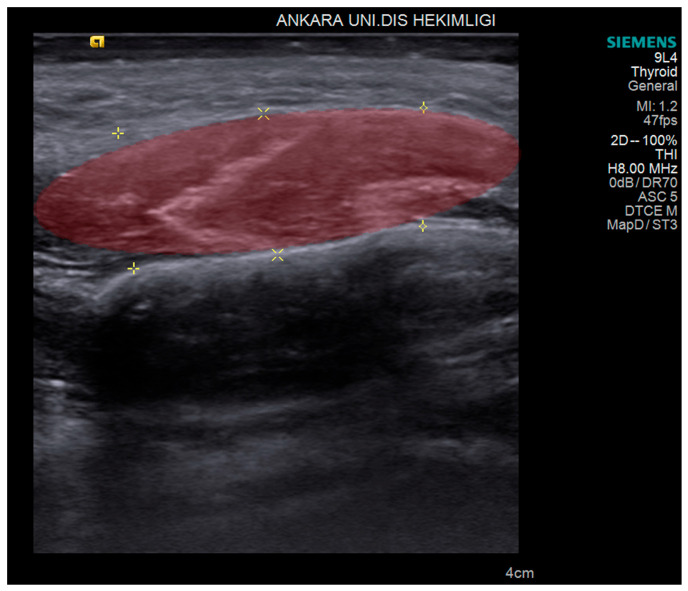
Volume of interest (red area) outlined on the ultrasonographic image of the masseter muscle that was used for machine learning and radiomics analysis. Yellow markers indicate the edge of masseter muscle.
